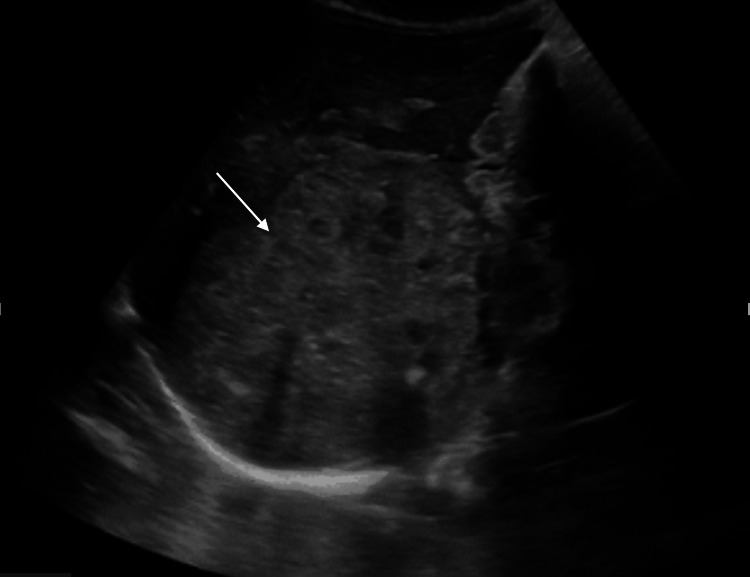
Abdominal right upper quadrant ultrasound showing soft tissue mass measuring 8.1 x 7.9 x 7.2 cm.
